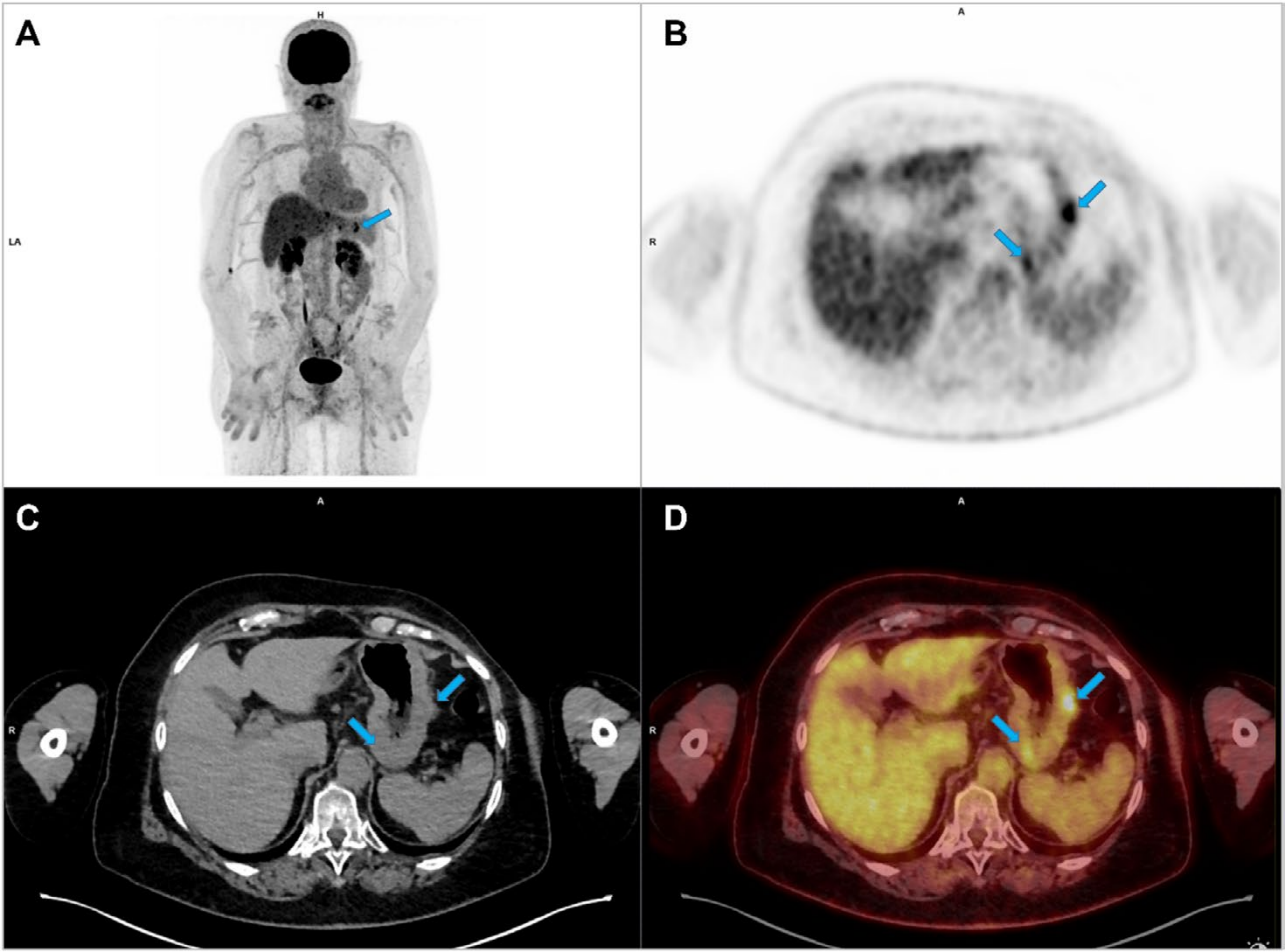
FDG PET/CT imaging showing a focal and high metabolism areas in the gastric region (blue arrows), SUVmax 6.3, on MIP (maximum‐intensity projection) images (A), axial PET slice (B) and axial fused PET/CT slice (C, D).
